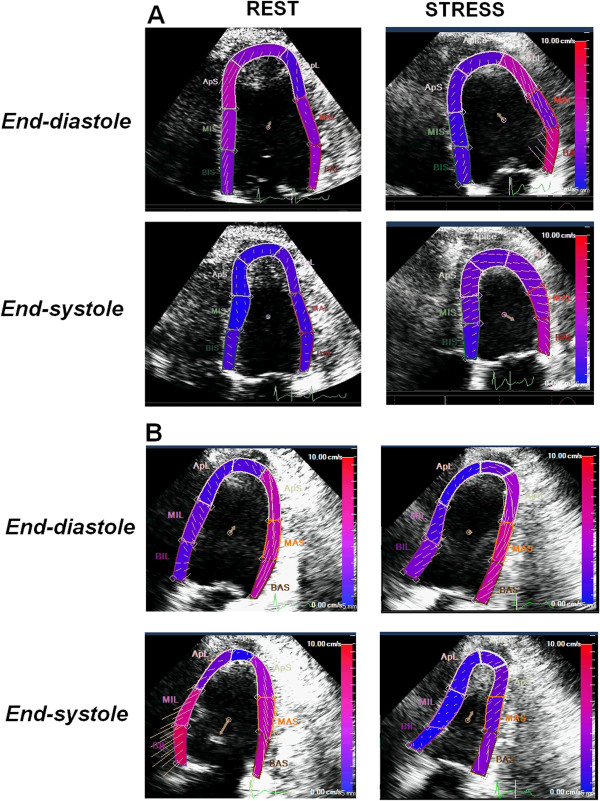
Donor #6 of Table 1. The same donor as in Figure 4. Two days after the subarachnoid hemorrhage and after brain death, the donor underwent a transthoracic dipyridamole stress echo (0.84 mg/kg in 6’) [see Additional file 4]. Left ventricular lateral wall hypokinesia and inferior wall akinesia are shown in 4-chamber (panel A) and 3-chamber (panel B) views at baseline (left panels). At peak stress a viability response is shown with recovery of lateral and inferior wall motion (right panels). The donor was considered suitable for heart donation and the heart was transplanted.
